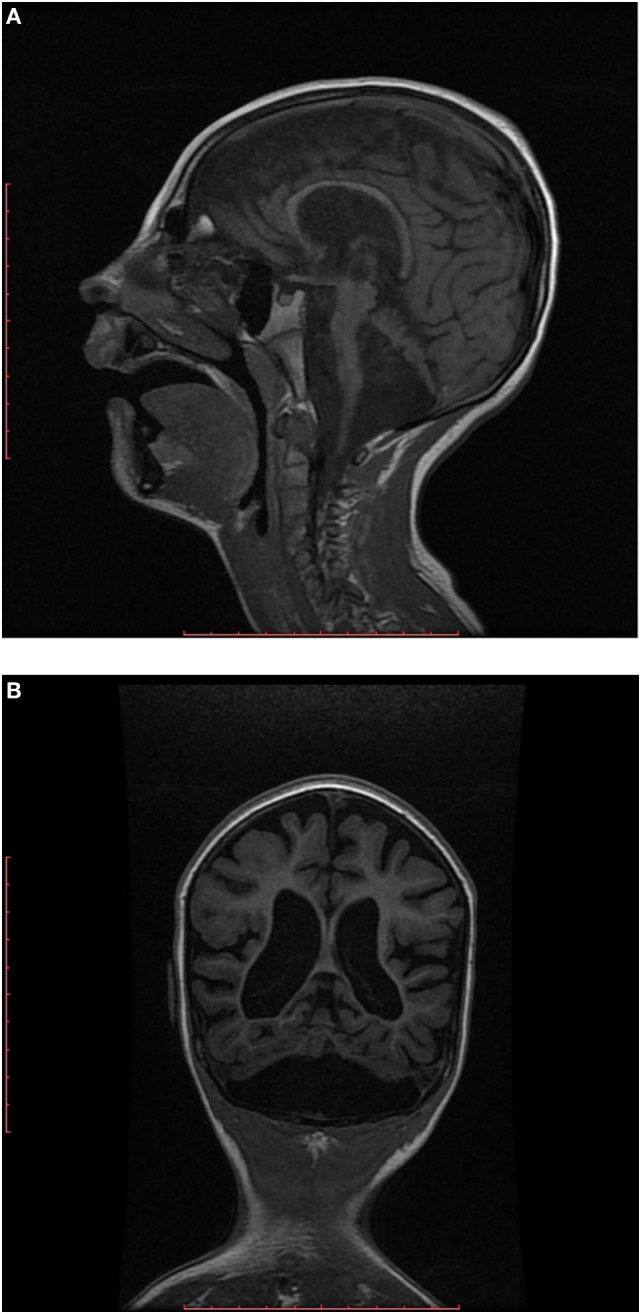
(A) Sagittal T1-weighted image shows hypoplastic cerebellum (cerebellar hemispheres more affected than vermis) associated with a small pons. (B) Coronal T1-weighted image shows markedly hypoplastic cerebellar hemispheres (dragonfly-like cerebellar pattern), with dilatation of the cisterna magna inferiorly and also cortical and cerebral atrophy.
